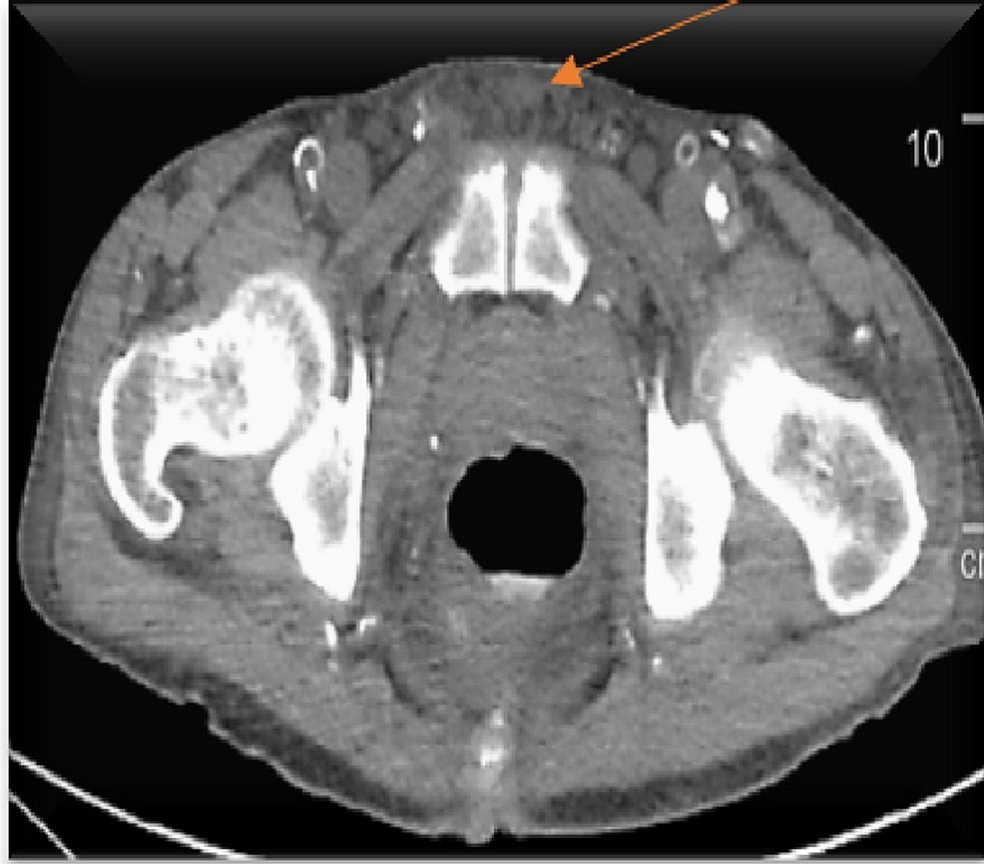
CT pelvic with fluid in the soft tissues.
